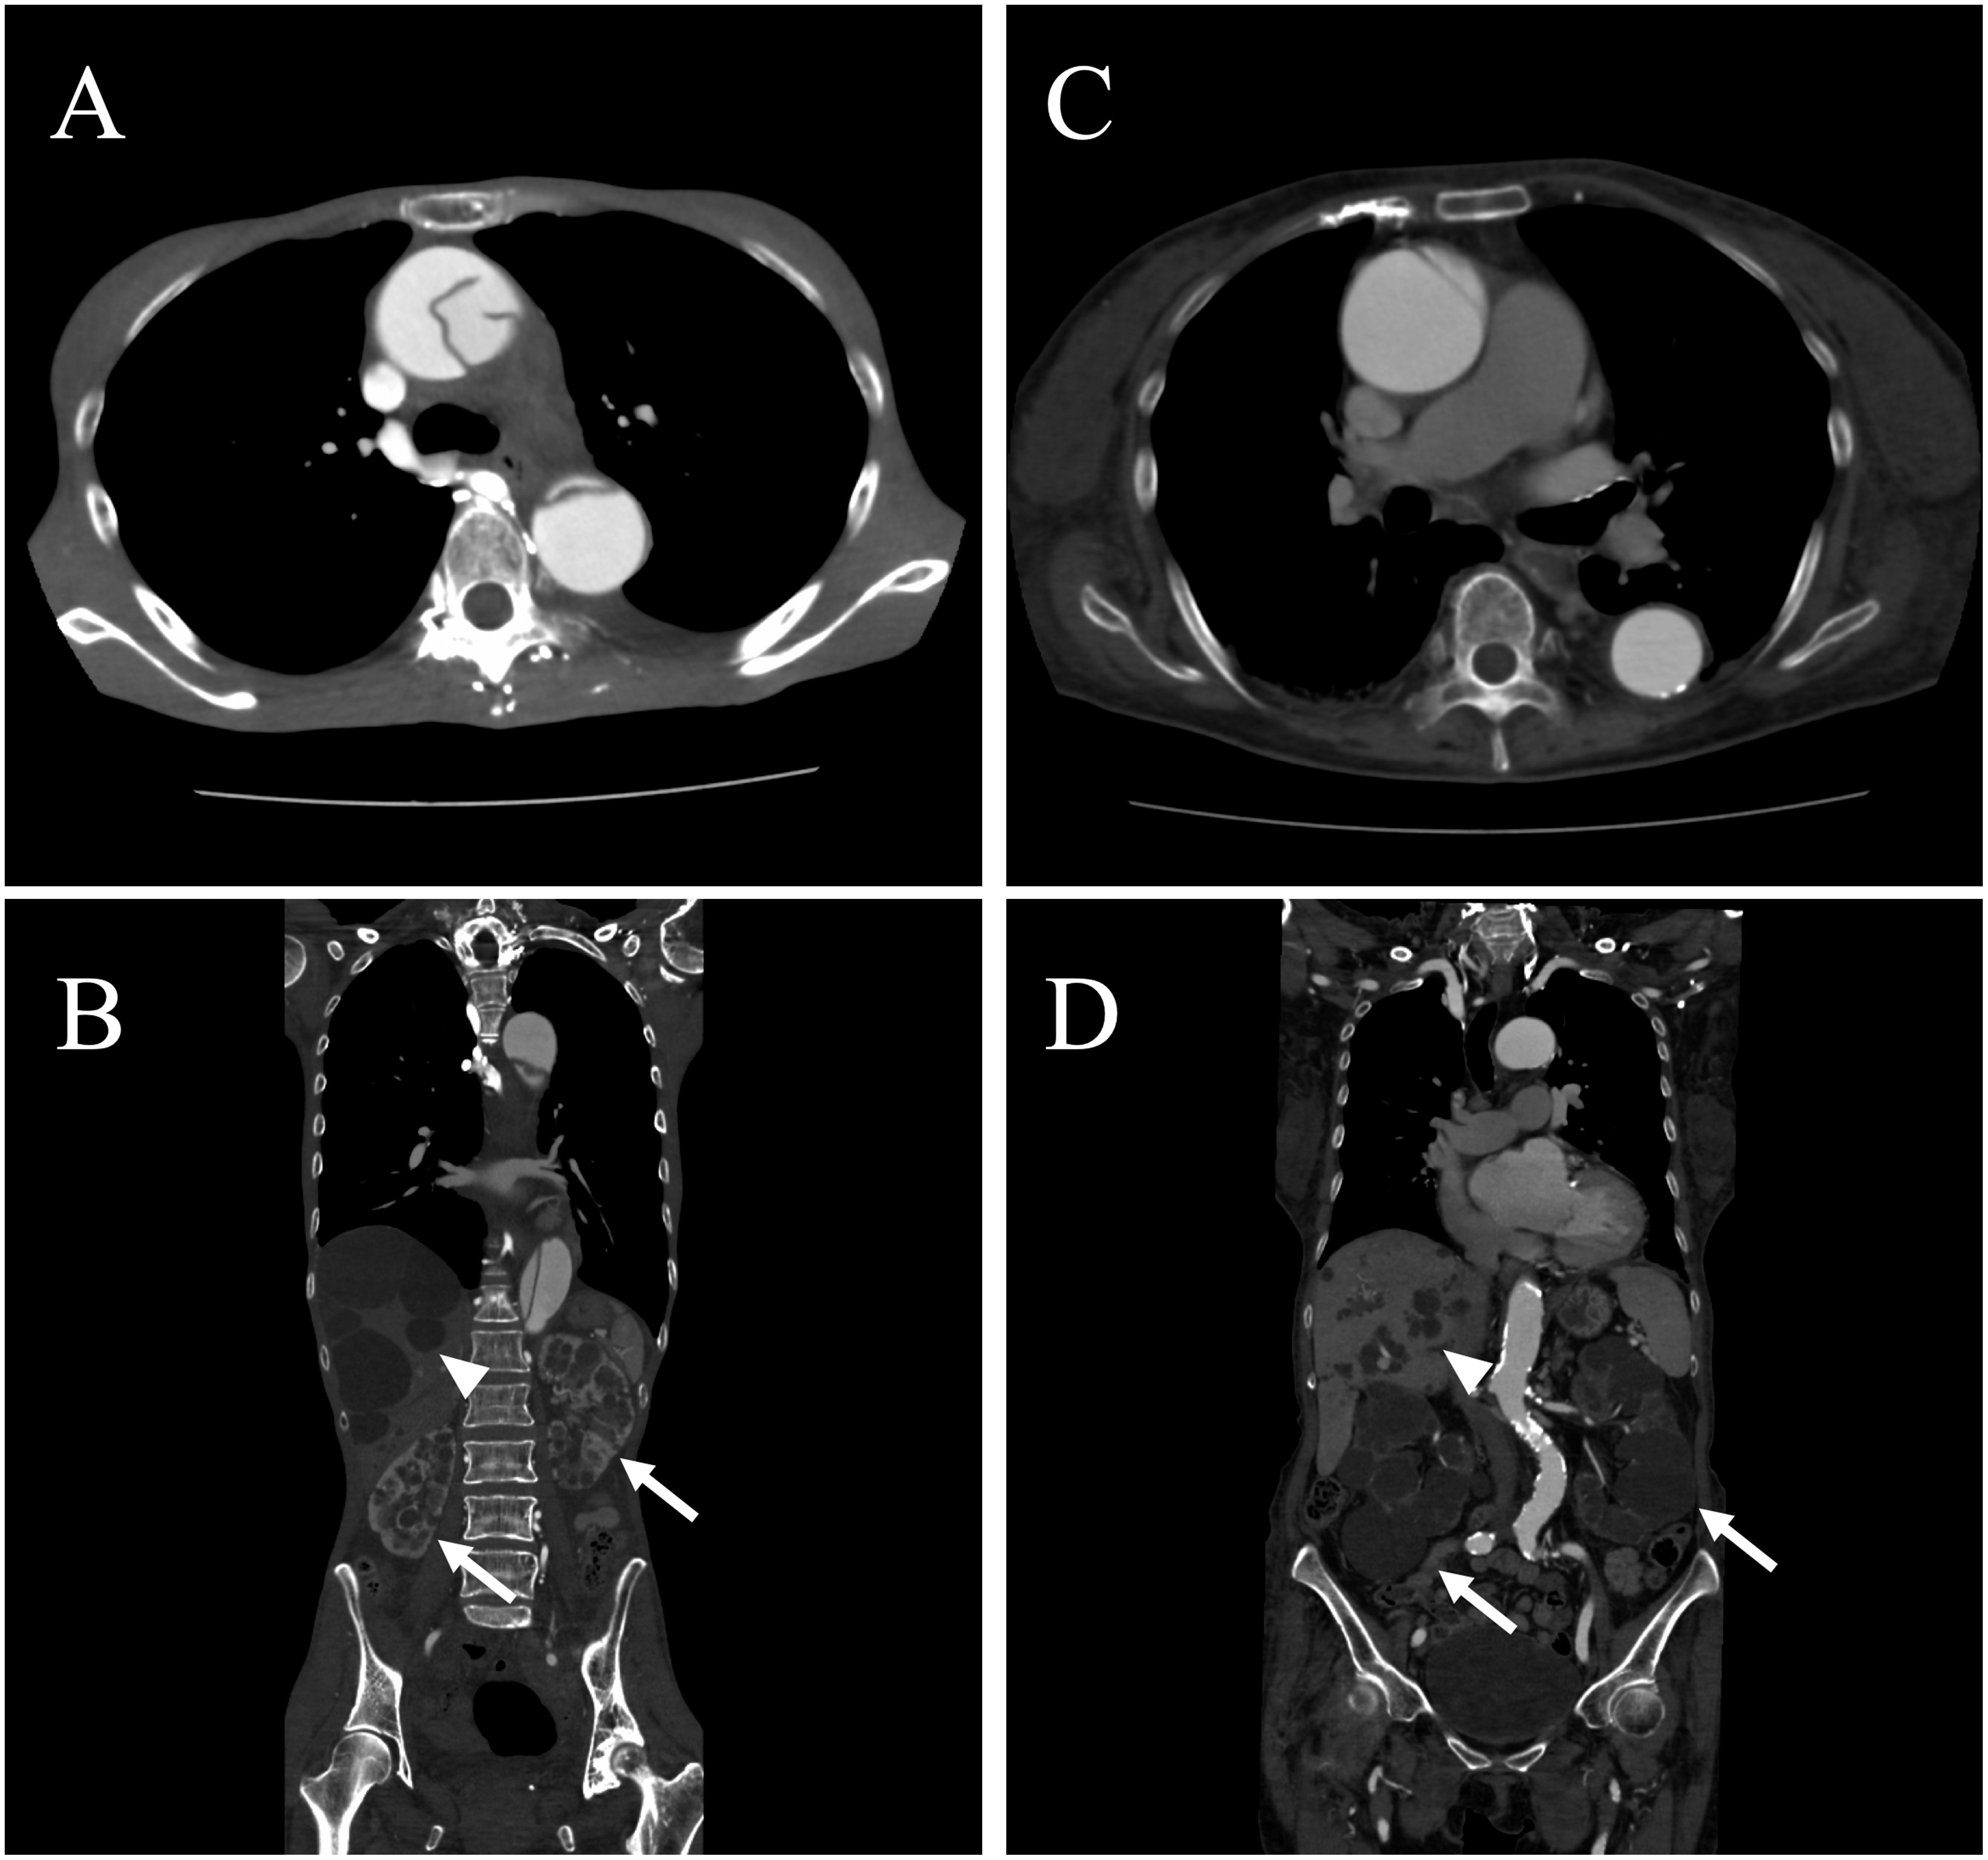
Fig. 1 Computed tomography of patients. Multiple renal cysts (white arrows) and liver cysts (white arrowheads) were detected. (A) Axial view of the daughter. (B) Coronal view of the daughter. A polycystic kidney was detected (white arrows). (C) Axial view of the mother. (D) Coronal view of the mother. A polycystic kidney was detected (white arrows).
